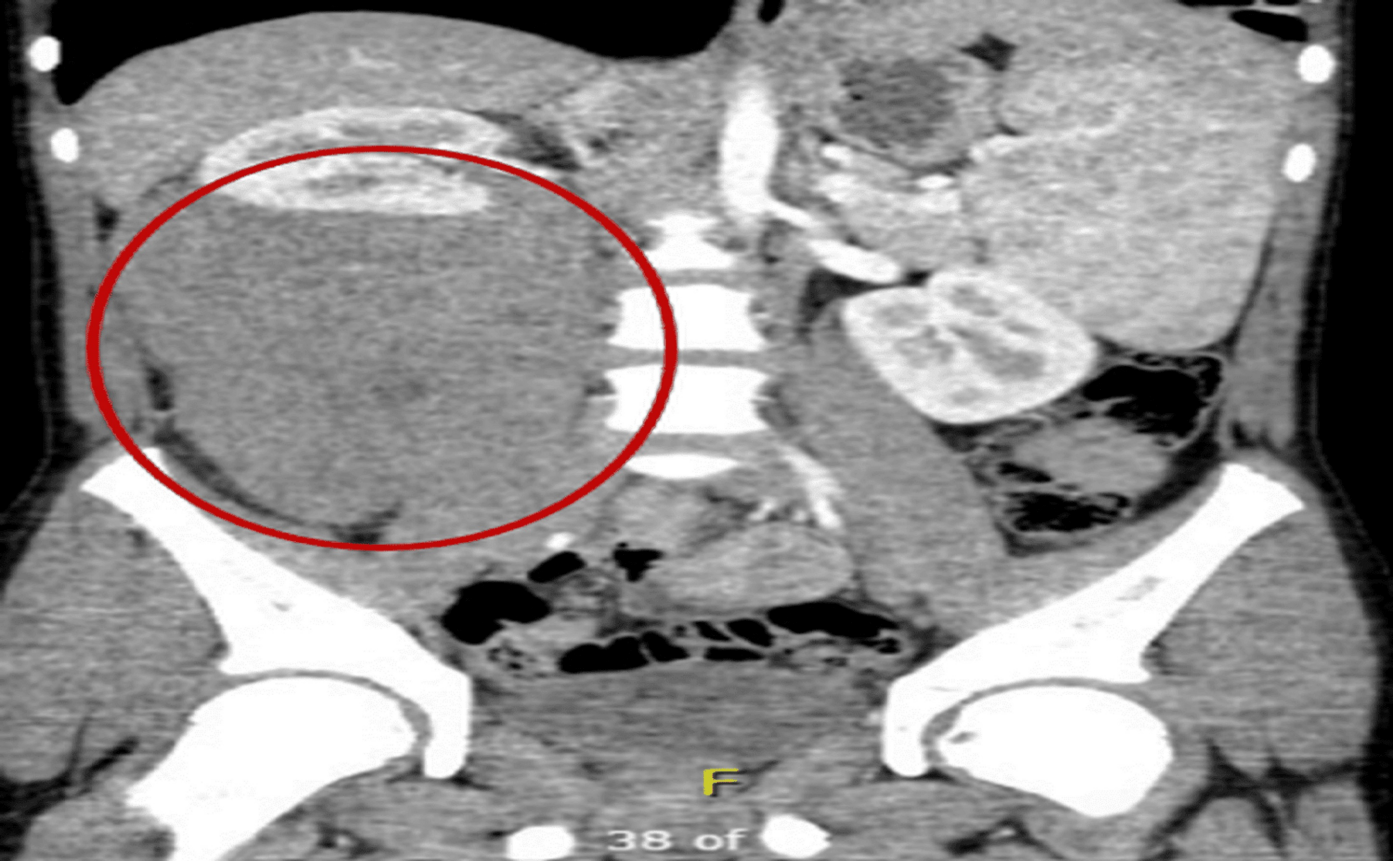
Contrast-enhanced abdominal and pelvic CT confirming a right perinephric mass. CT: computed tomography
